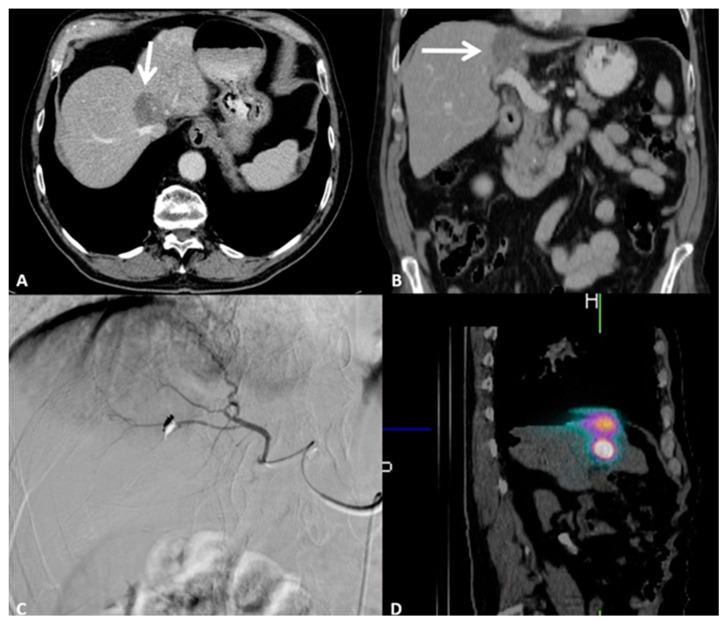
79-year-old male patient presenting with pain in the right upper abdominal quadrant due to intrahepatic cholangiocellular carcinoma. Performance Status One, no surgical indication; the patient underwent eight cycles of chemotherapy. (A) Contrast-enhanced axial CT scan showing a 3.5 cm lesion of the I-IV segment adjacent to the inferior vena cava and to the right hepatic vein. (B) Contrast-enhanced coronal CT scan showing how the lesion is adjacent to the portal vein. (C) Digital subtraction angiography runs showing super selective catheterization of the target vessel with intra-arterial administration of marked albumin macroaggregates, which serve as the diagnostic phase of radioembolization. (D) Single-photon emission computed tomography oblique reconstruction scan showing focal and selective uptake of marked albumin macroaggregates by the target lesion, confirming the catheter position for the therapeutic radioembolization.
